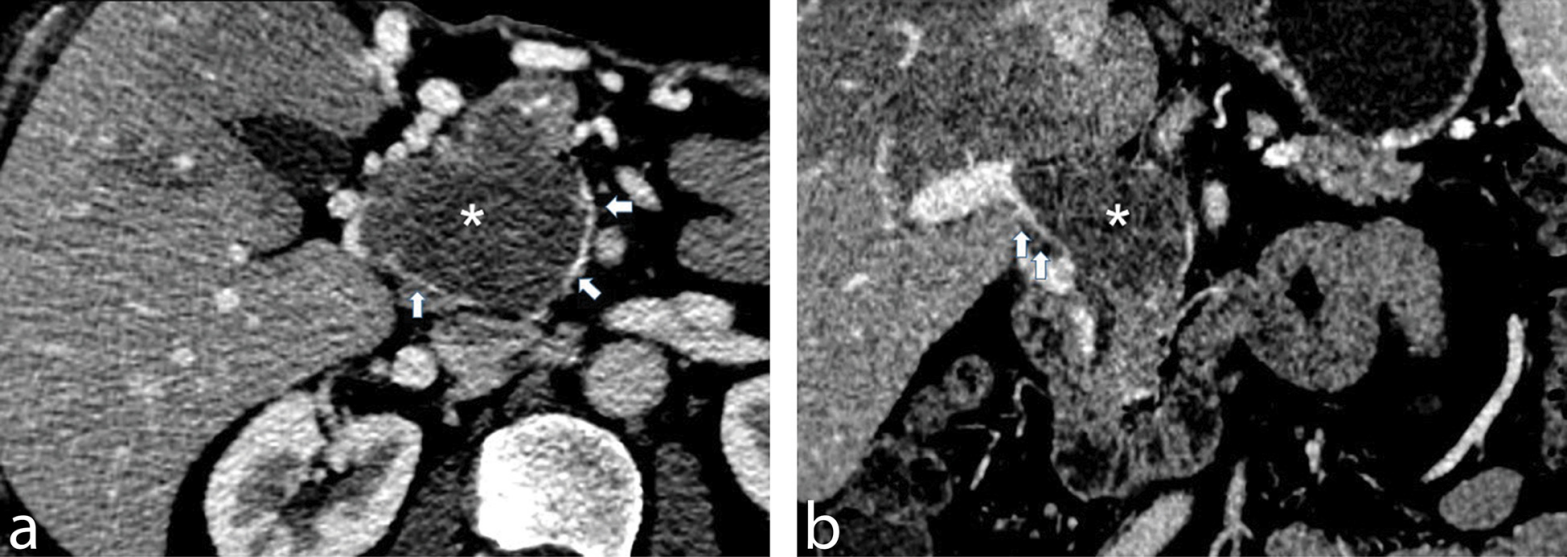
Multiplanar contrast-enhanced multidetector CT images reveal a mass in the portal vein (*). Axial CT image (a) shows numerous collaterals around the heterogeneously enhancing mass (*). There is a rim of contrast around the mass (white arrowheads) indicating that the mass has an intraluminal origin rather than tumour invasion from adjacent structures. Corresponding coronal CT image (b) shows a sharp interface with the appearance of a “beak” (white arrowheads) between the opacified main portal vein and the mass (*).
